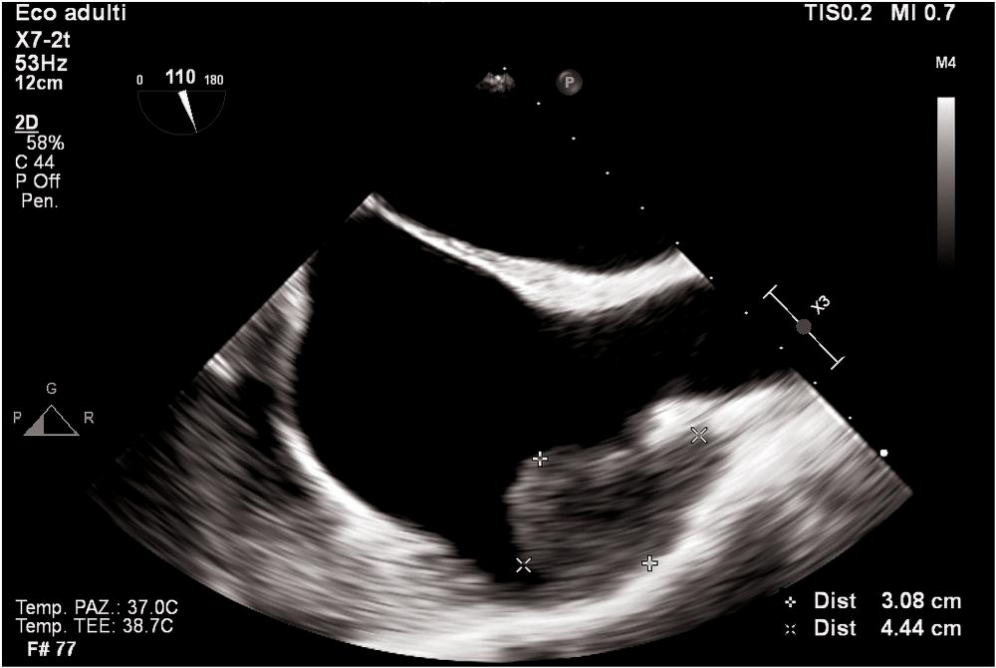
Transoesophageal echocardiogram mid-oesophageal view at 90° (bicaval view). The figure shows a mass in the right atrial appendage.
